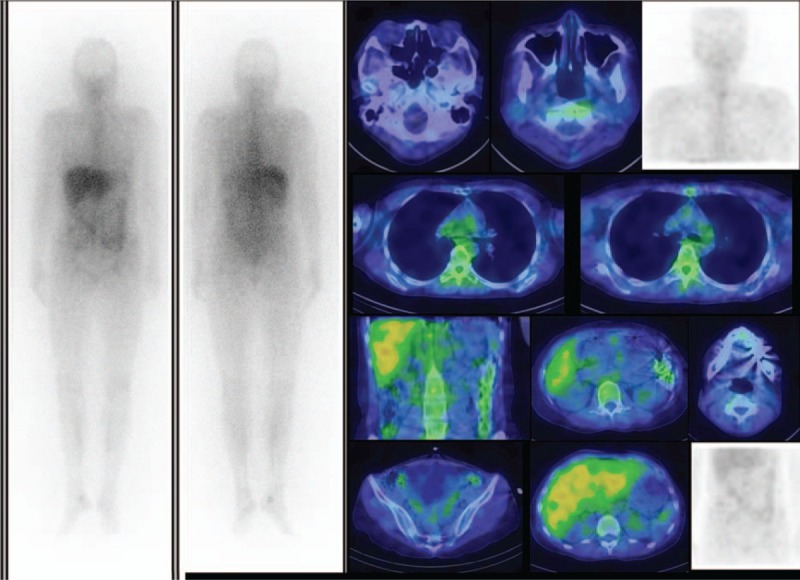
Three months after treatment, gallium scan with SPECT/CT only showed physiological uptake; the abnormal radioactive foci identified on the prior scan were no longer present.
